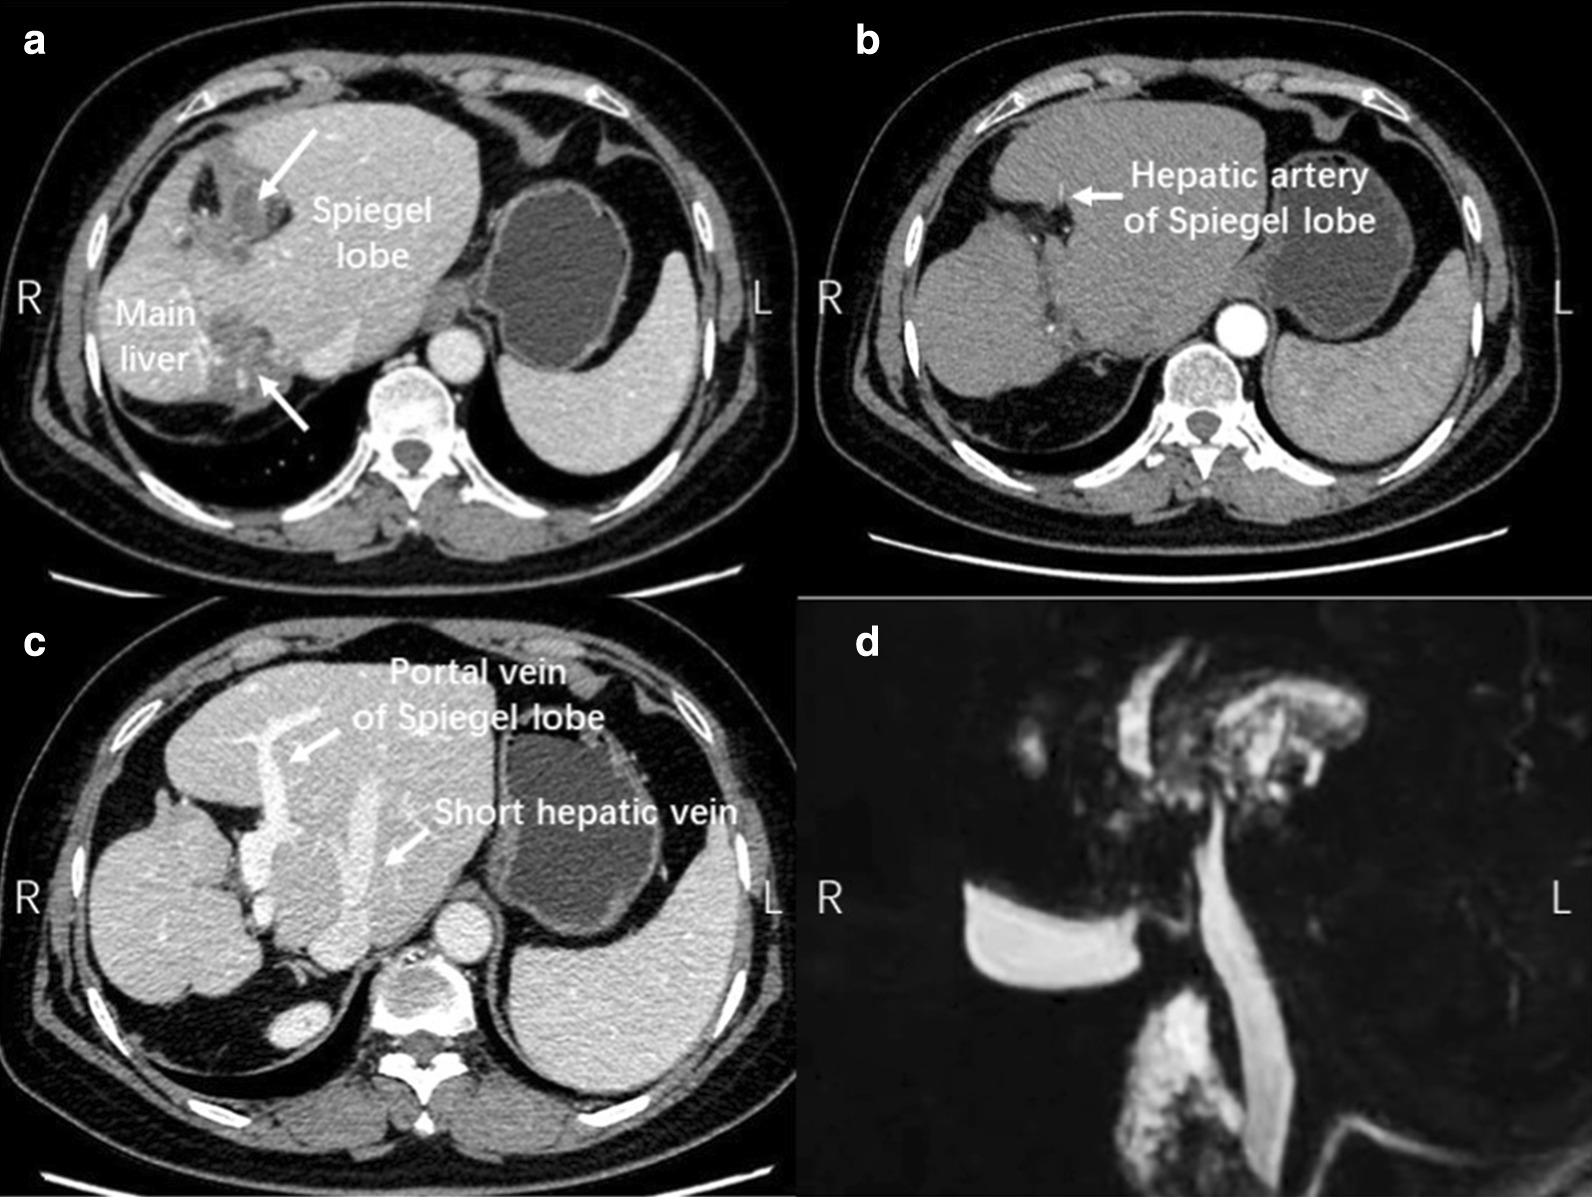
Imaging assessment of case 1. a CT image showed hepatolithiasis in the right liver and left liver (arrow). b Contrast CT image showed hepatic artery of Spiegel lobe. c Contrast CT image showed hepatic vein and portal vein of Spiegel lobe of the caudate lobe. d MRCP showed no stricture in extrahepatic bile duct
